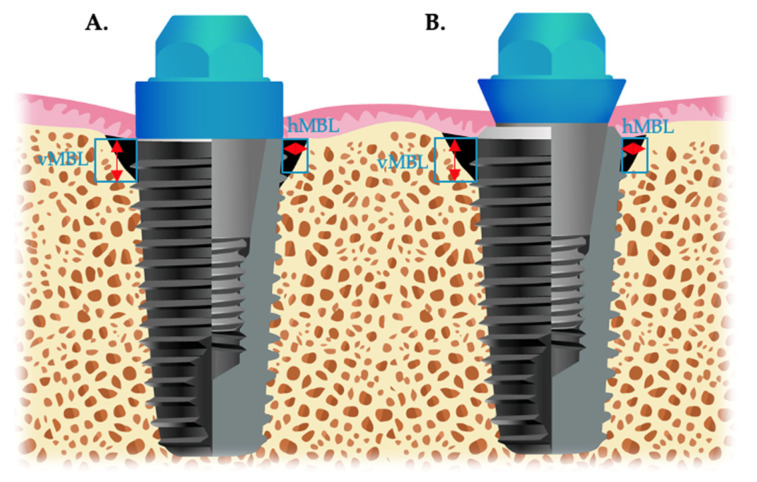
Radiographic measurements of the platform-switched and platform-matched dental implants. (A) Platform-matched dental implants (PM). (B) Platform-switched dental implants (PS). vMBL, vertical marginal bone level; hMBL, horizontal marginal bone level.
